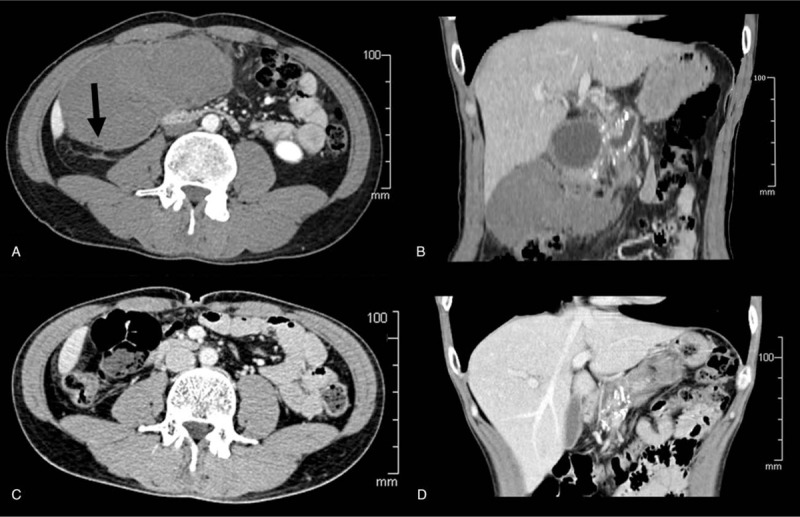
(A) The emergent computerized tomography (CT) scan shows a large mesenteric hematoma with the size of 8.2 cm × 11.7 cm in front of the head of pancreas in the right abdominal cavity. Gas in the hematoma (arrow) verifies the hematoma comes from mesentery. (B) CT scan reveals the large mesenteric hematoma with the size of 8.2 cm × 11.7 cm in front of the head of pancreas in the right abdominal cavity. (C) CT scan shows the large mesenteric hematoma has completely absorbed after 3 months. (D) CT scan reveals the large mesenteric hematoma has completely absorbed, the large pancreatic pseudocyst has disappeared and the pancreatic stones have decreased significantly. CT = computed tomography.
